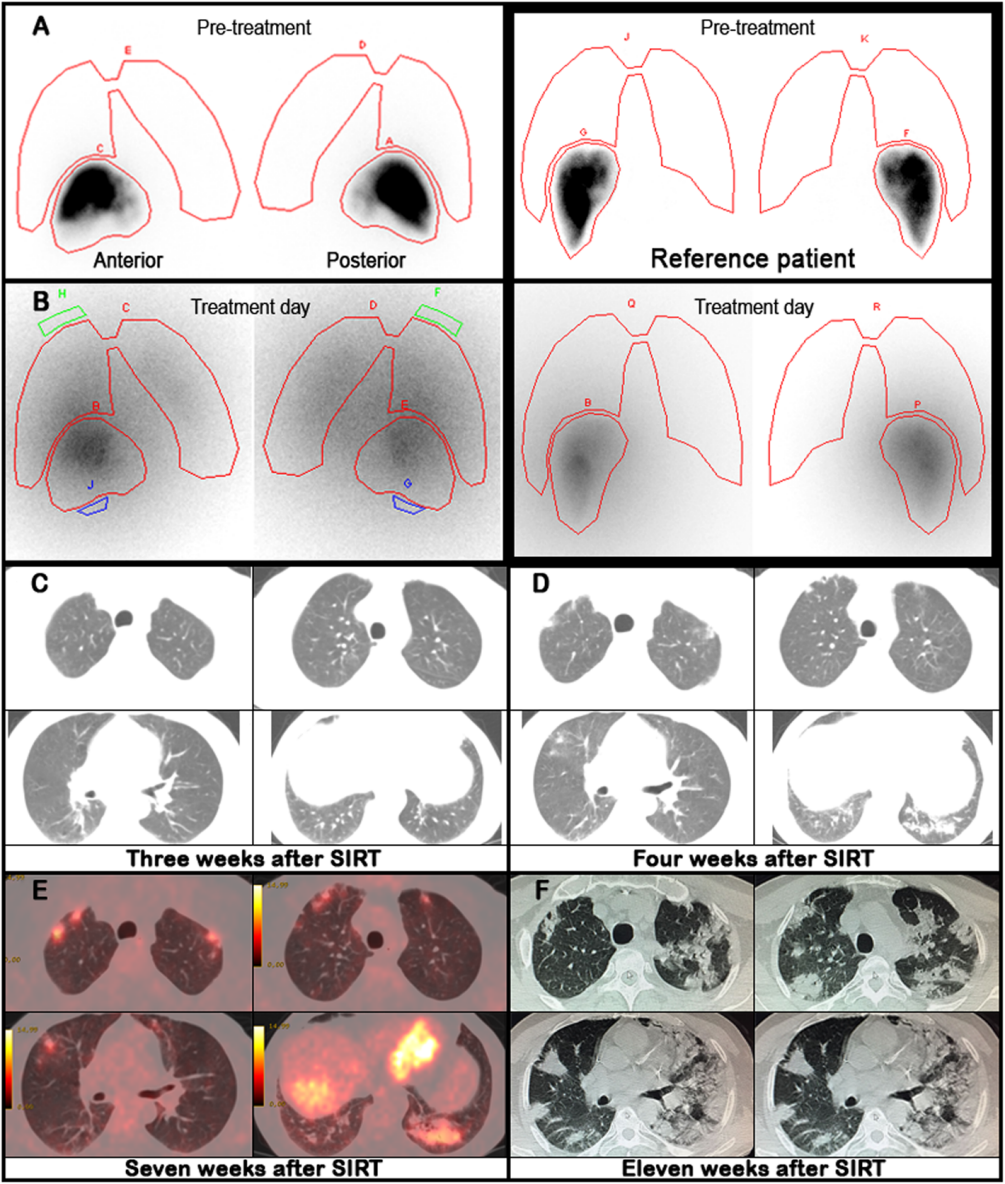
Comparison of the planar scintigraphic images of the case (1a, 1b) and reference patient for liver-lung shunt imaging and transaxial fluorine-18 fluorodeoxyglucose positron emission tomography (18F-FDG PET/CT- 1e 1E) / computed tomography (CT- 1c, 1d, 1f) images obtained after selective internal radiation therapy (SIRT)
